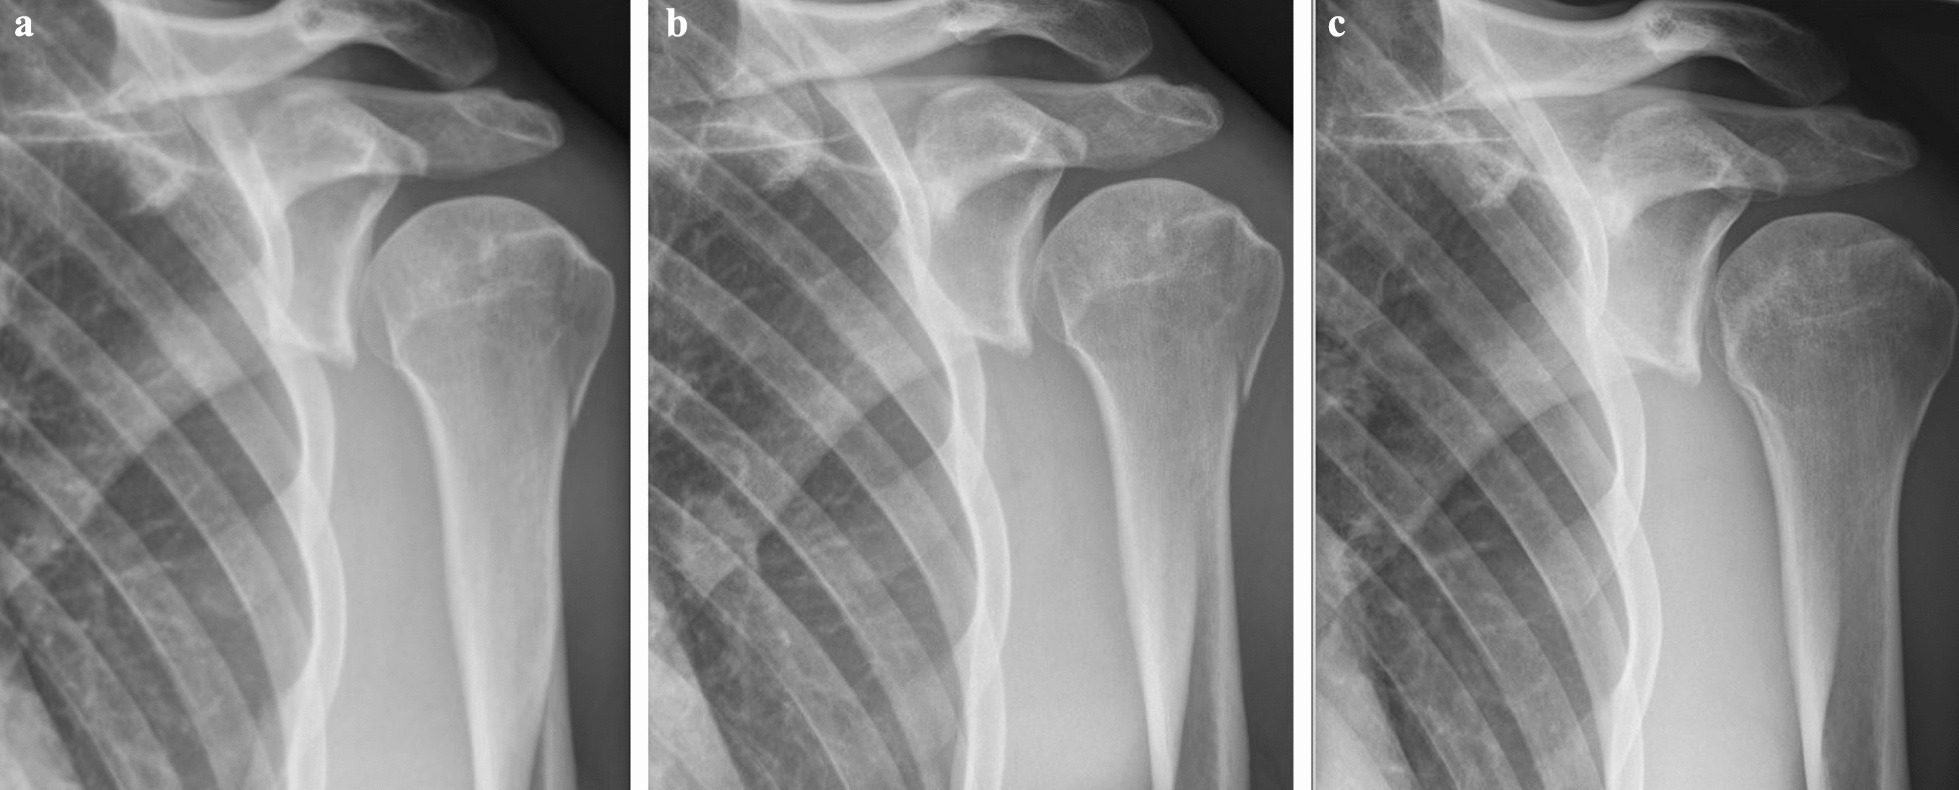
Radiographic pictures of the delayed union fracture and the healing process 18 days after the start of the treatment (a); 34 days between first and second picture (b); 18 days between second and third picture (c)
